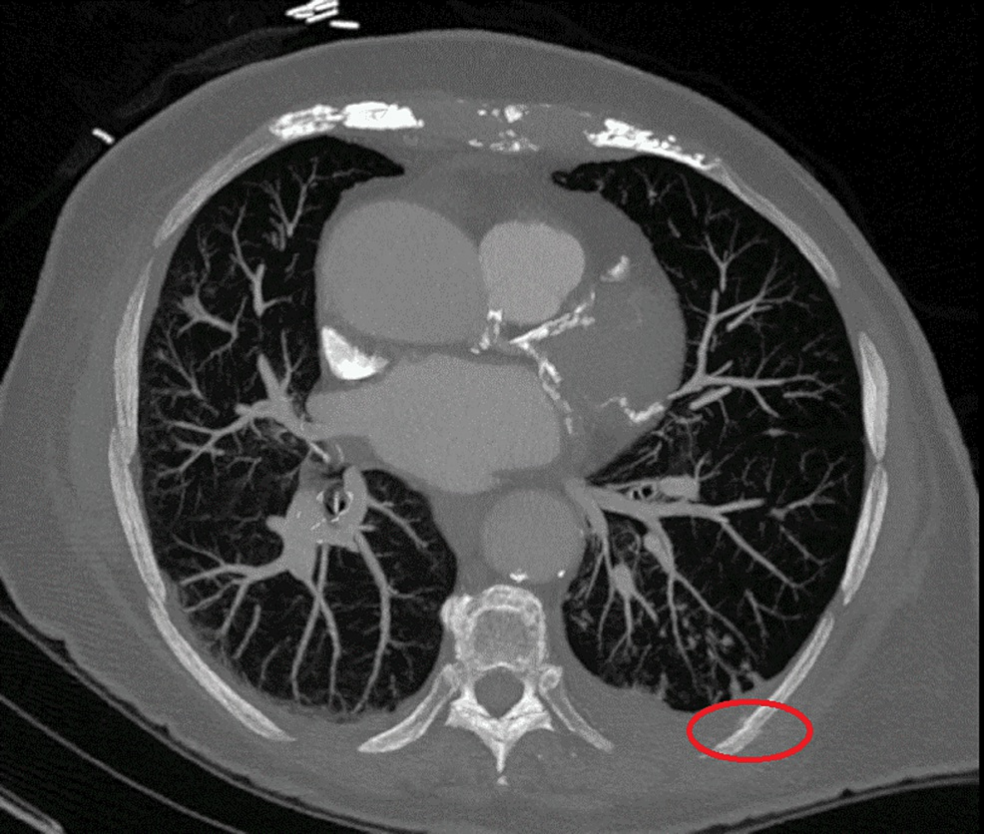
High-resolution coronal CT image demonstrating fracture at left rib 8.
